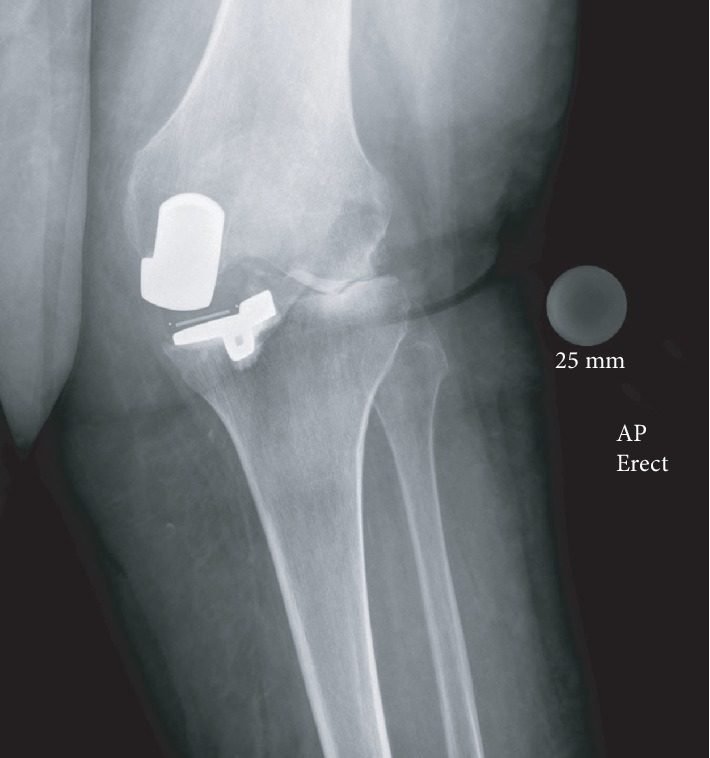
Weight-bearing AP radiograph of the left knee. Medial unicompartmental knee replacement in situ. Loss of lateral joint space, subchondral sclerosis, and infarction of the lateral femoral condyle.
